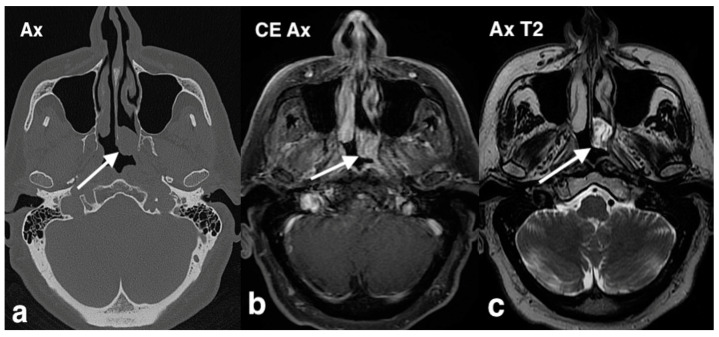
Inverted papilloma in an 83-year-old woman presenting with right nasal obstruction for 2 years associated with fronto-temporal headache and epistaxis. Endoscopically, a lobulated mass protruding in the left nasal cavity was found. The axial CT image (a) shows a mass (arrow) located in the posterior third of the left nasal cavity, between the nasal septum and medial pterygoid plate, and protruding posteriorly through the left choana in the nasopharynx. No bony erosion is visible. On MRI, in the T1 axial fat saturation image after intravenous Gd (b), the mass shows intense heterogeneous enhancement, with typical cerebroid pattern (arrow); on the T2 axial image, the mass shows inhomogeneous, cerebroid aspect, with high intensity, alternating hypointense and hyperintense striation throughout the mass (arrow) (c).
